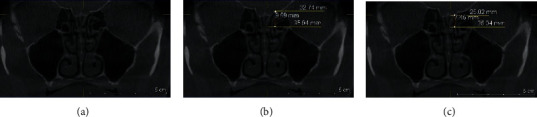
(a) Coronal CBCT demonstrating type 3 TMS classification on the left side. (b) OFL-ER measurement and (c) OFL-CP measurement coronal plan of CBCT.
